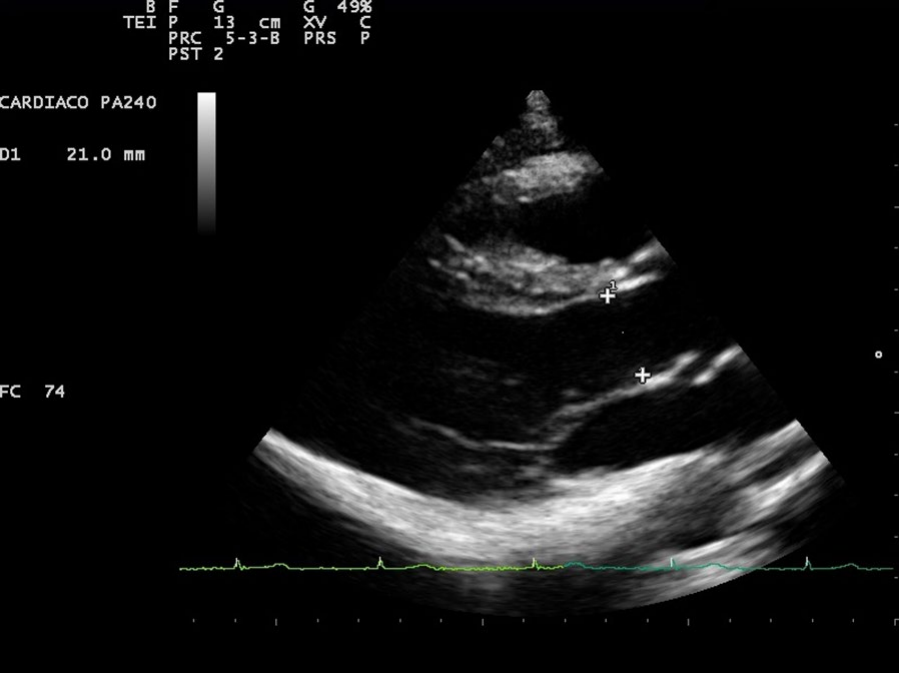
Measurement of the LVOTd from a parasternal long-axis view
